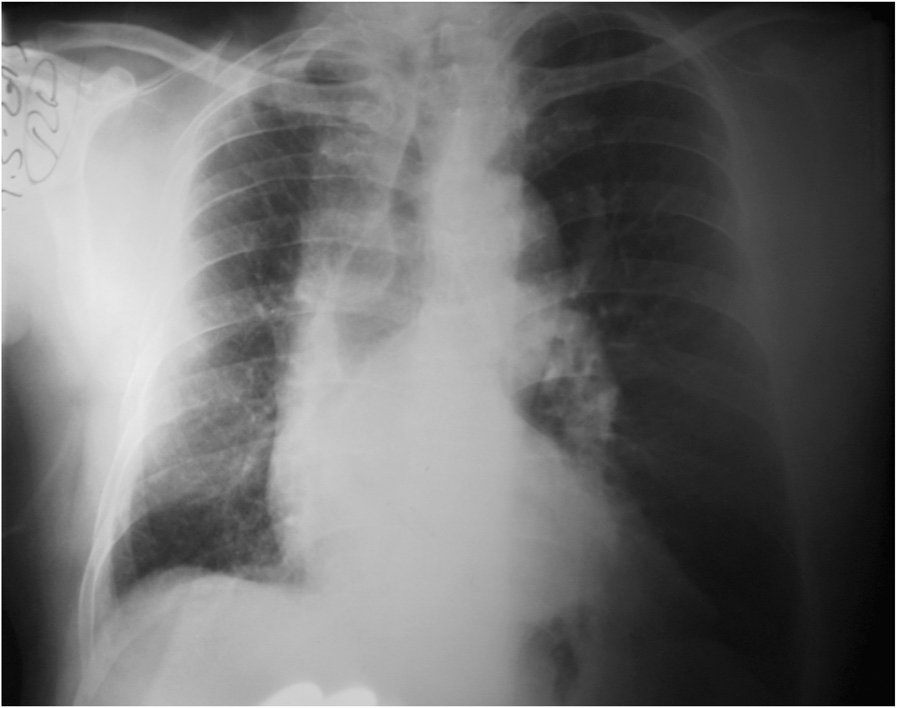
Chest x-ray showing prominent left pulmonary artery with congested hyperinflated left lung and shifting of the mediastinum to the right side
